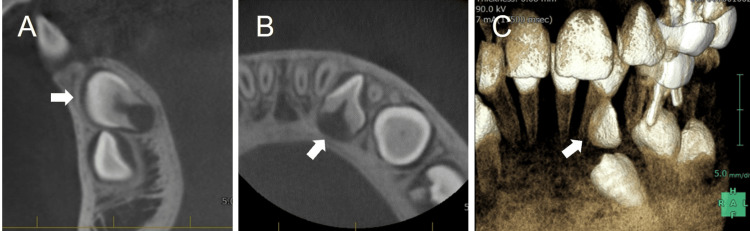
Cone-beam computed tomography images obtained at the age of 8 years 3 months. Arrows indicate the supernumerary tooth. (A) Sagittal section. (B) Horizontal section. (C) Three-dimensional construction image.
